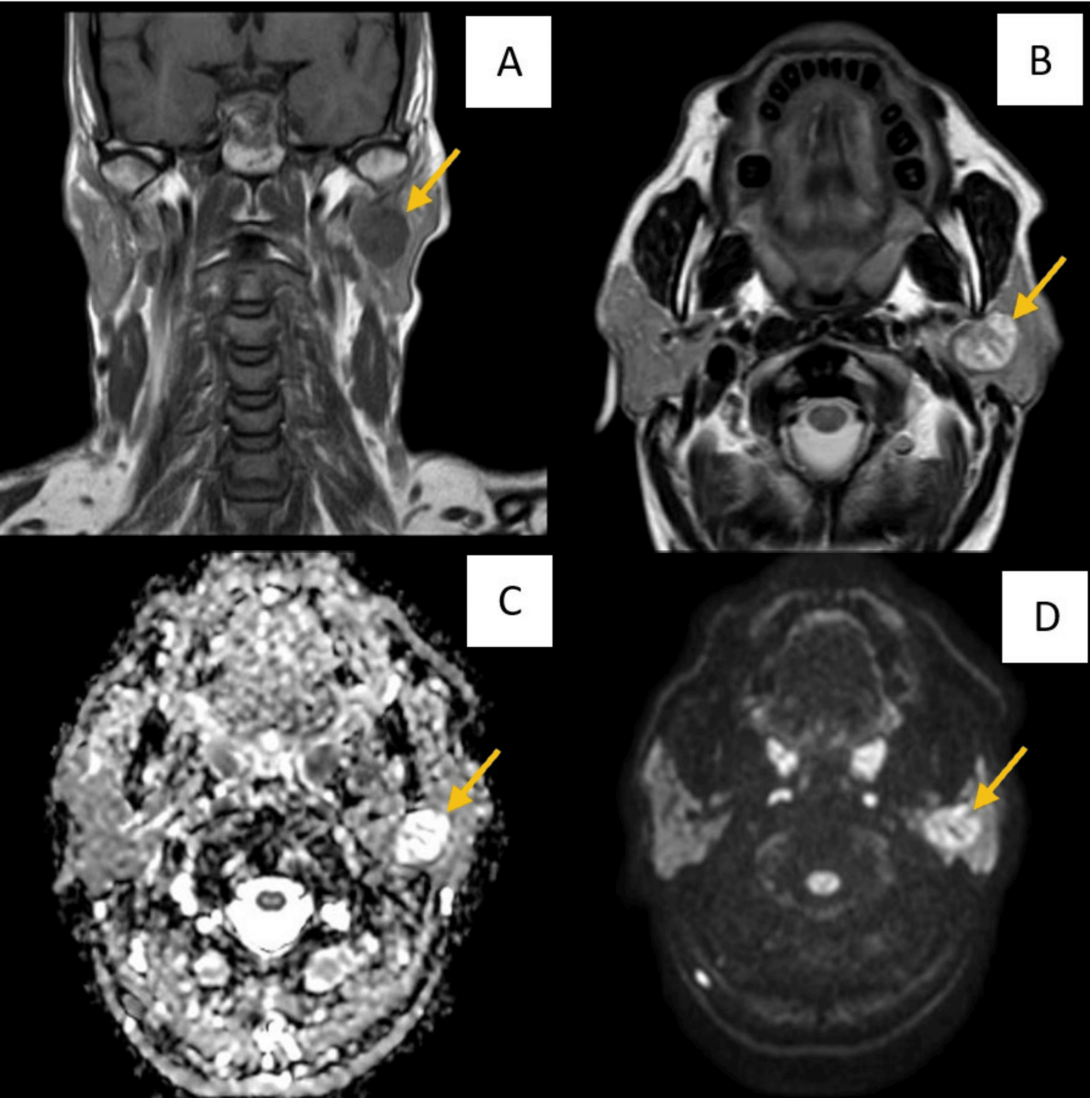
MRI images of a 55-year-old male showing primary benign neoplastic etiology involving the left parotid gland - likely Warthin's tumor A: T1-weighted imaging MRI sequence showing a well-defined hypo-intense lesion, with a lobulated margin seen in the superficial and deep lobes of the left parotid gland; B: T2-weighted imaging MRI sequence showing a well-defined hyper-intense lesion, with a lobulated margin seen in the superficial and deep lobes of the left parotid gland; C and D: ADC and DWI images showing subtle peripheral diffusion restriction
